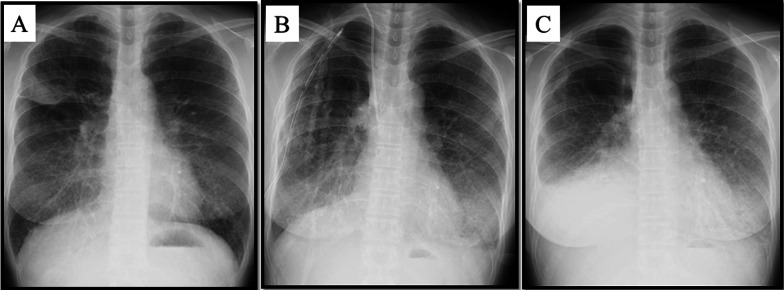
Chest radiograph findings of complications after thoracic surgery in a representative LAM patient (Case 1 in Table 2). a Preoperative chest radiograph shows aspergilloma in the right upper lung field. b Chest radiography findings 2 days after right upper lobectomy. A drain was inserted into the right thoracic cavity, and a central venous catheter inserted in the right internal jugular vein. Both are visible in the right lung field. This figure shows decreased radiolucency in bilateral lungs and pleural effusion on the opposite side of the surgery. c A chest radiograph 14 days after surgery shows that pulmonary congestion and pleural effusion are getting worse
